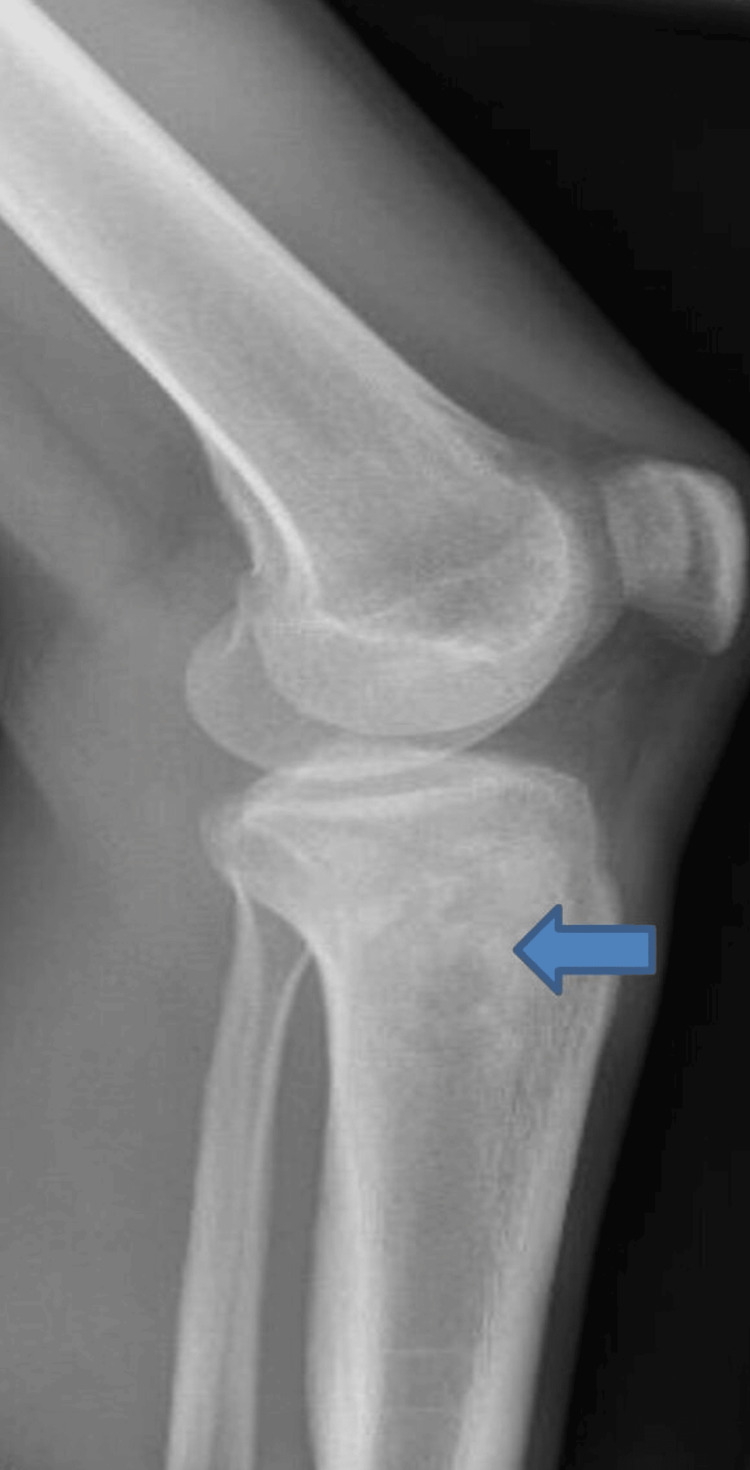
X-ray of the left knee showing lytic lesion (Blue arrow)
